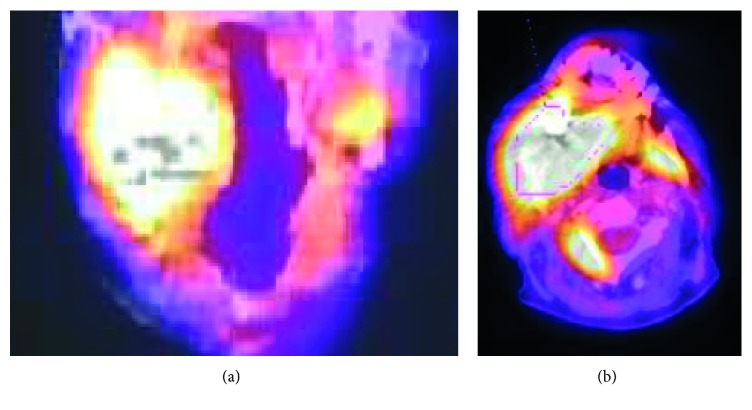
PET/CT of the patient's mandibular mass. This image shows an avidly hypermetabolic mass of the right mandibular angle. (a) Coronal cuts; (b) axial cuts.
